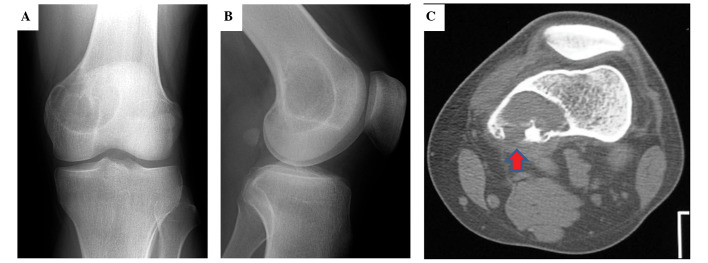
(A) Anteroposterior and (B) lateral radiographs of the distal femur tumor. An eccentric osteolytic lesion is visible in the medial condyle; however, there is no apparent periosteal reaction. (C) Axial computed tomography demonstrates a distinct lesion with (size, 48×45×30 mm) and destruction of the posterior cortex (red arrow).
